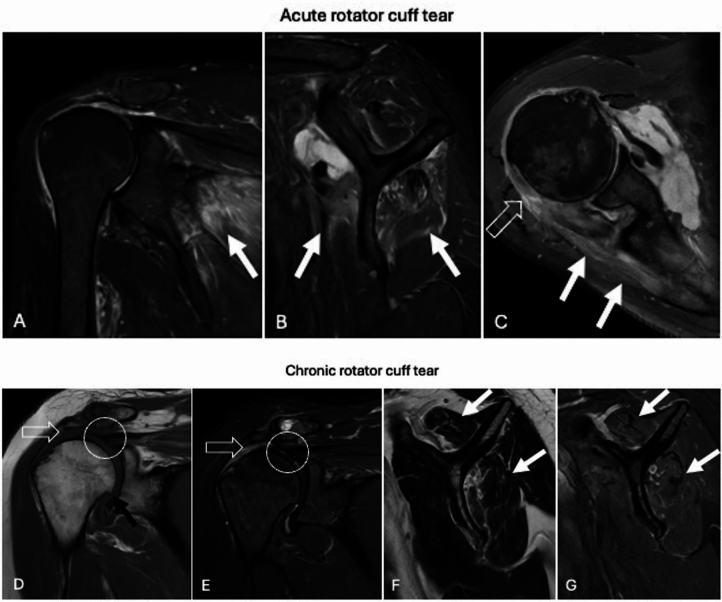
Acute rotator cuff tear. Shoulder MRI performed 6 days after trauma with fat-suppressed proton density weighted images (A, B, C) show edematous and frayed retracted infraspinatus tendon (C, void arrow), intra- and peri-muscular edema (A, B, C; arrows). Muscle trophism is well-preserved and the glenohumeral joint does not show degenerative changes. Chronic rotator cuff tear. Shoulder MRI performed eleven months after trauma show complete supraspinatus tendon tear with massive retraction of the tendon that appear rounded, atrophic, and hypointense (circle) on coronal T1-weighted (D) and fat-suppressed proton density weighted image (E). Mild atrophy and fat infiltration (white arrows) without inter- and/or peri-muscular edema can be noted in supraspinatus and infraspinatus muscles on sagittal T2-weighted (F) and fat-suppressed proton density weighted (G) images. Glenohumeral osteoarthritis (D; black arrow), humeral cranialization and reduction of the subacromial space (void arrows) are observed
